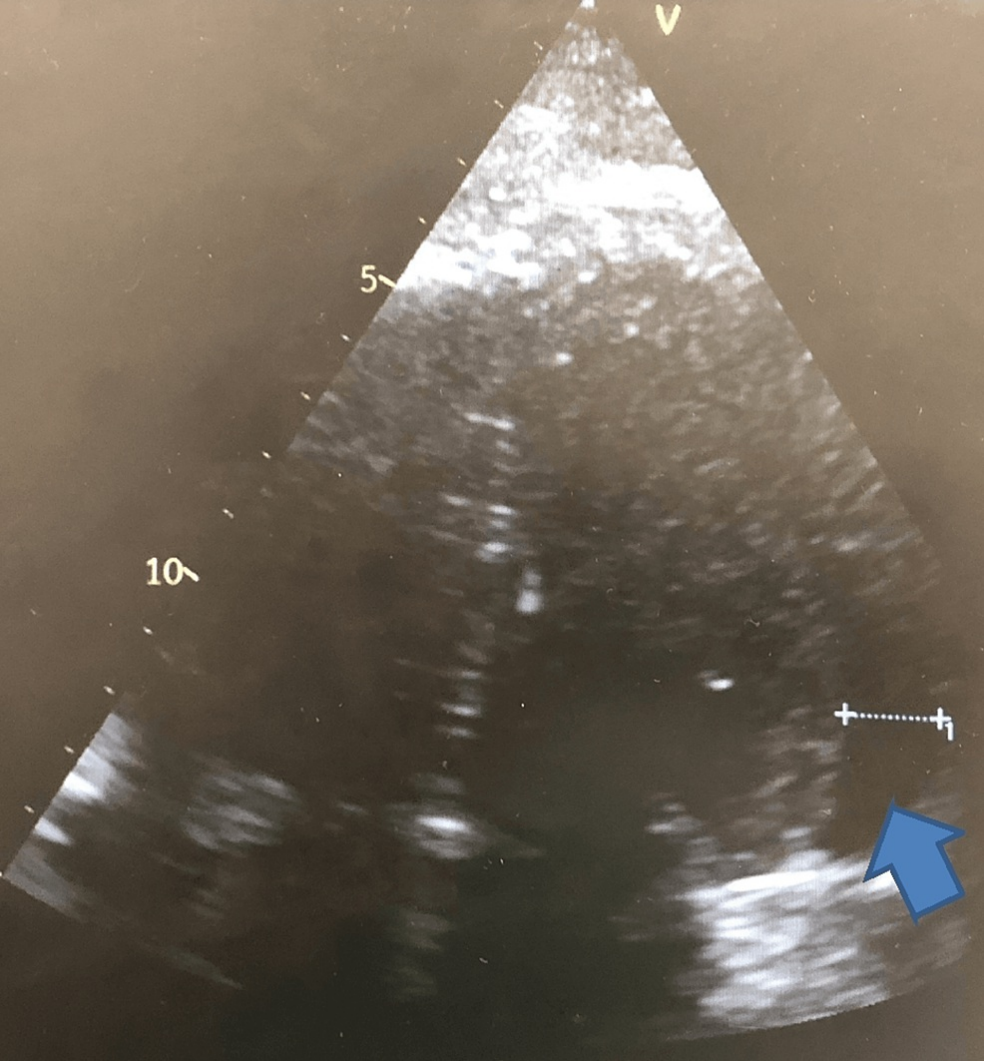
Apical 4 chamber echocardiographic view showing pericardial effusion (pointed blue arrow)
